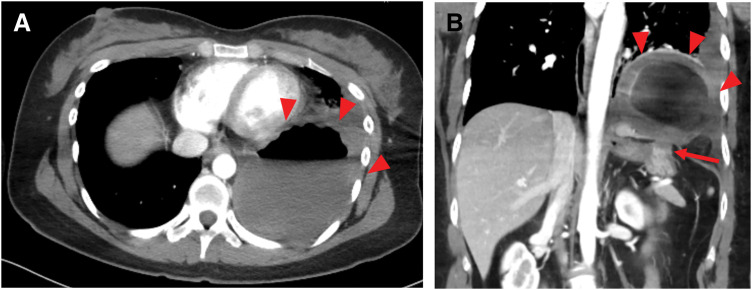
Representative images of contrast-enhanced CT at the first visit. (A) Axial image. The arrowheads indicate the incarcerated gastric fundus, which was visualized as a cavitary lesion with an air–fluid level in the thoracic cavity. (B) Coronal image. The arrowheads indicate the incarcerated gastric fundus; the arrow indicates the hernial orifice. The incarcerated fundus was visualized as a cavitary lesion in the thoracic cavity and was continuous with the stomach in the abdominal cavity at the hernial orifice. It exhibited poor contrast.
